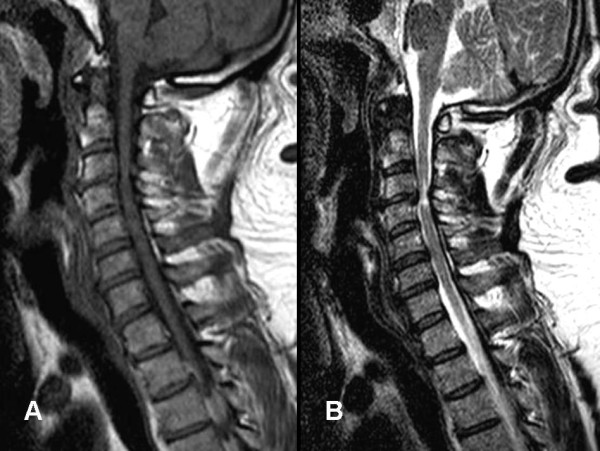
Magnetic resonance imaging (MRI) of the cervical spine shows cervical spinal canal stenosis at the C3-4 segment. (A) T1-weighted MRI image without contrast. (B) T2-weighted MRI image. The T2-weighed sagittal image shows intramedullary hyperintensity and no signs of spinal cord swelling. The images are compatible with chronic spondylotic cervical myelopathy.
